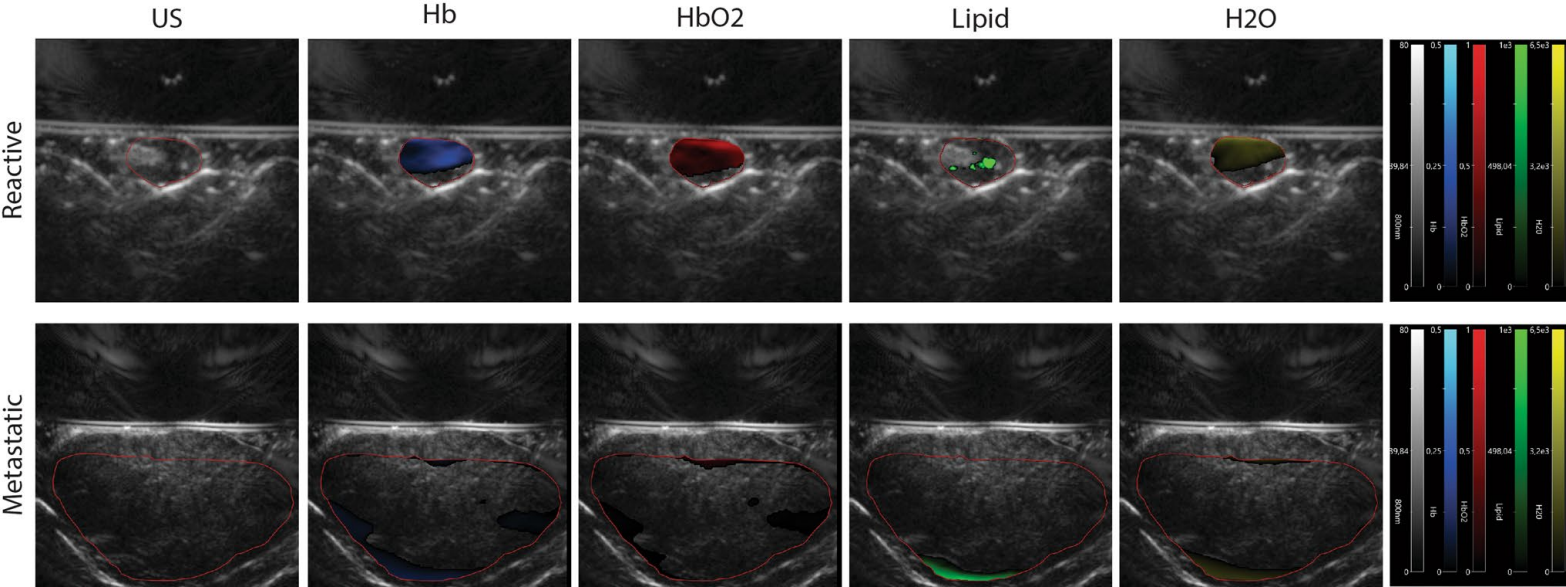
Visualization of the unmixed chromophores (Hb, HbO_2_, lipid, water) in a reactive and a metastatic lymph node. Region of interest shown in ultrasound image (left column)
